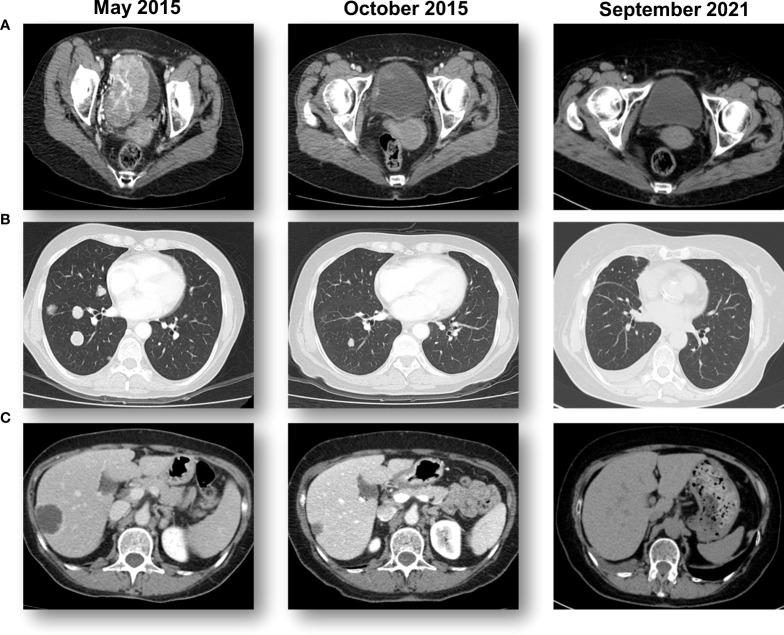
Sequential CT scans showing a response to pemprolizumab. (A) A large bladder tumor that was biopsied prior to starting pembrolizumab immunotherapy with a complete response in the bladder. (B) Multiple lung metastases from bladder cancer that obtained a complete response with pembrolizumab immunotherapy. (C) Multiple hepatic metastases from bladder cancer that obtained a complete response with pembrolizumab immunotherapy.
